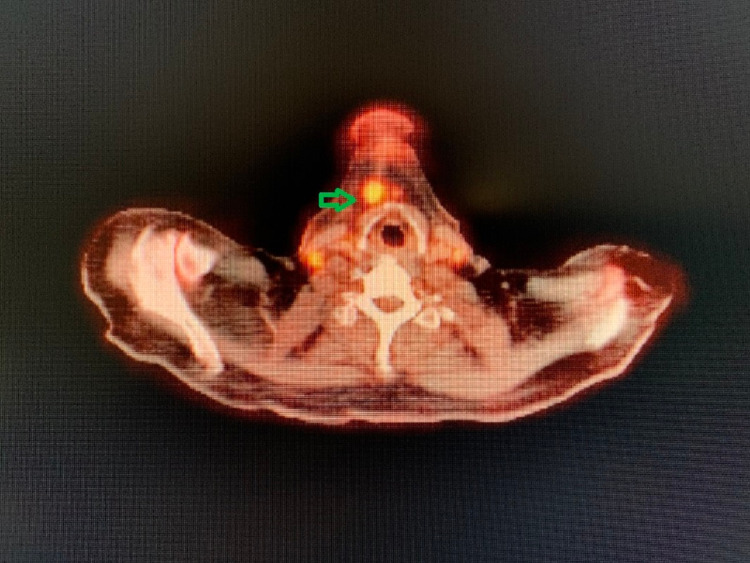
PET scan showing submental lymhadenopathy FDG uptake is seen in the right submental lymph node FDG, fluorodeoxyglucose
